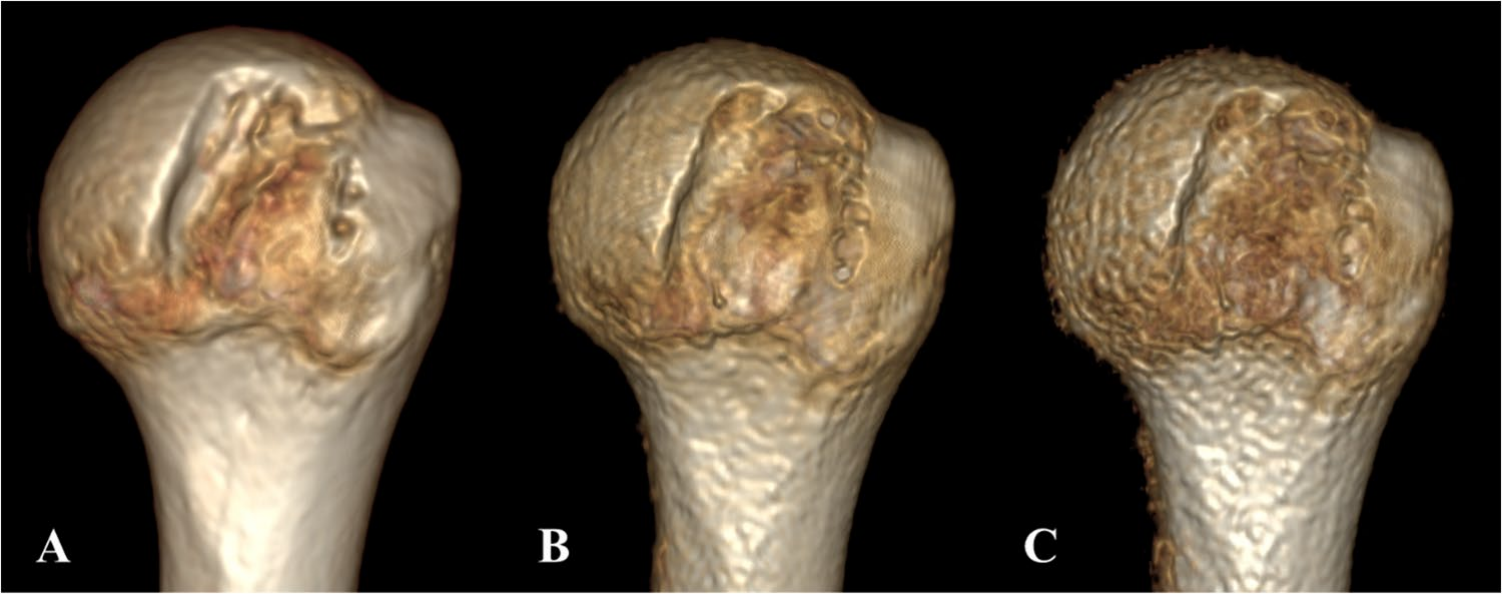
Comparison of 3D VR of the humerus. CT (A), DL ZTE (B), and conventional ZTE (C)
